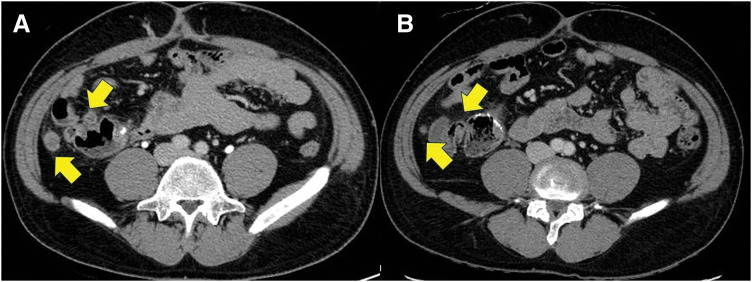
Imaging findings before and after chemotherapy. Yellow arrows shows a disseminated nodule and a swelling lymph node. (A) Follow-up CT after initiation of EP therapy revealed peritoneal dissemination nodules adjacent to the anastomosis site and enlargement of the surrounding lymph nodes. (B) CT after 6 courses of Bmab/FOLFOXIRI revealed a marked reduction in both the peritoneal dissemination nodules and lymph node enlargement. Bmab, bevacizumab; EP, etoposide and cisplatin
